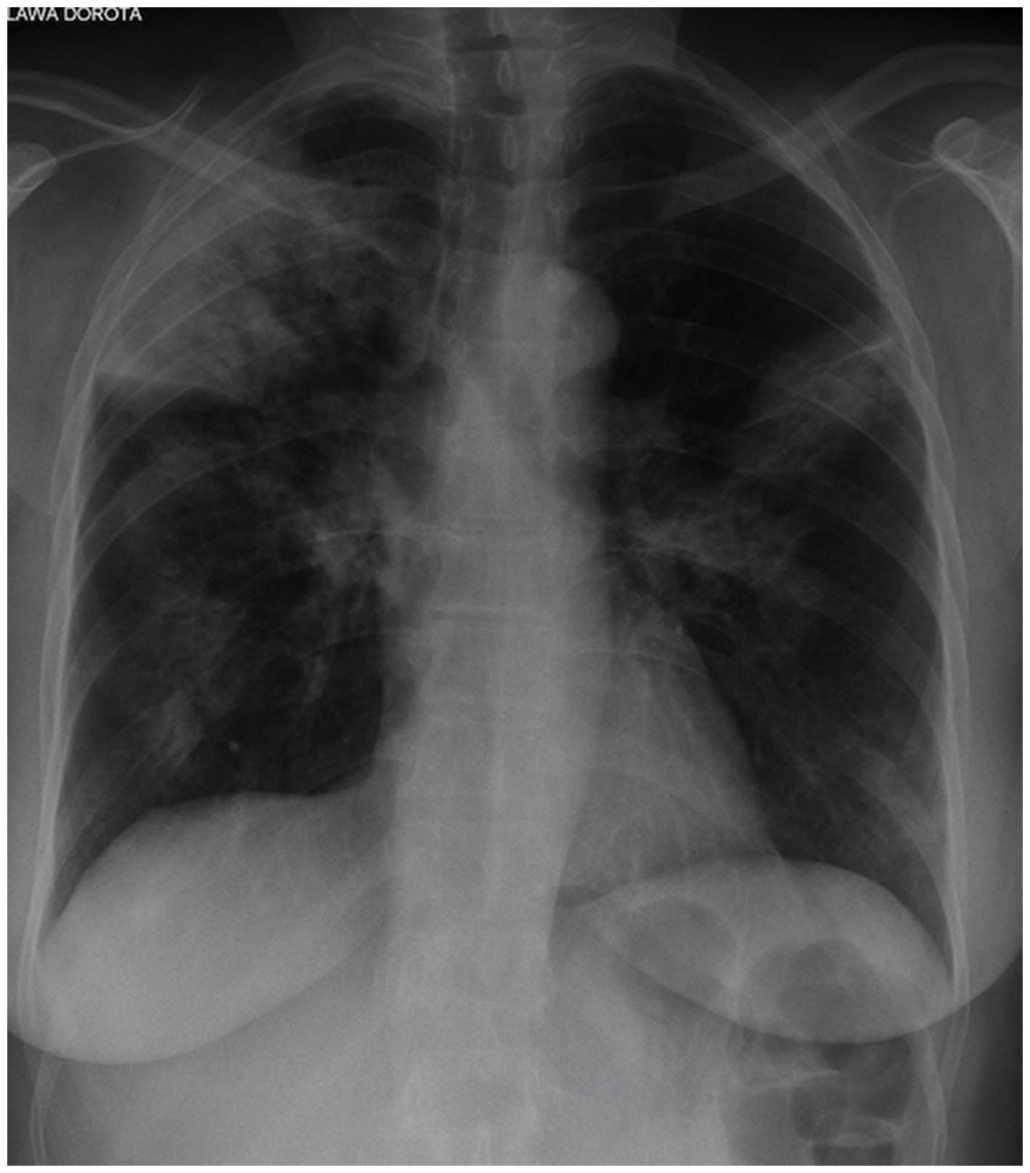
Chest X-ray of OP. Bilateral opacities localized in the peripheral parts of the both lungs.
